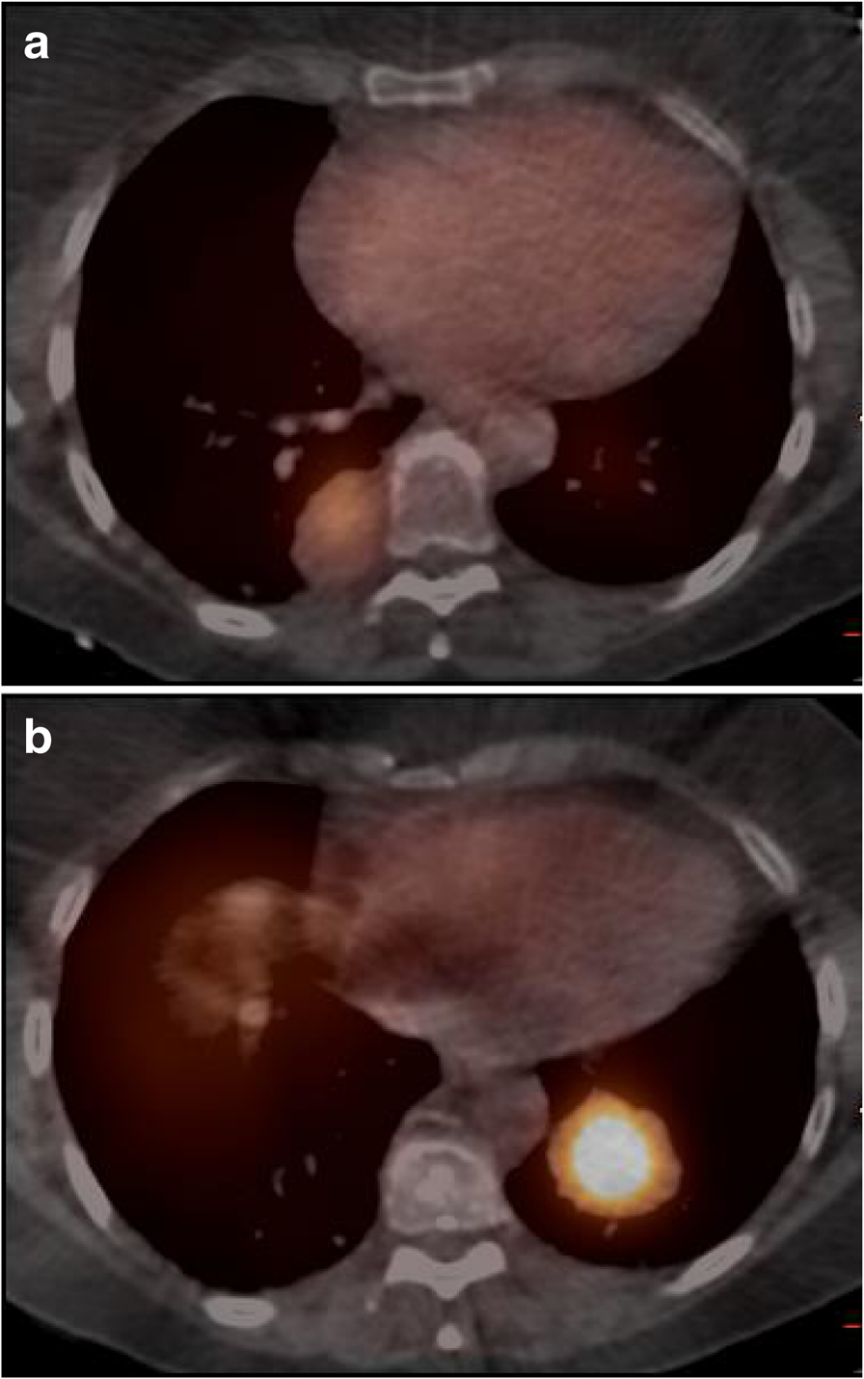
^111^In-OTSA-101 SPECT-CT images performed at H72 in patient 3 showing a grade 2 lesion in the right lower lung lobe (a) and a grade 4 lesion in the left lower lung lobe (b)
